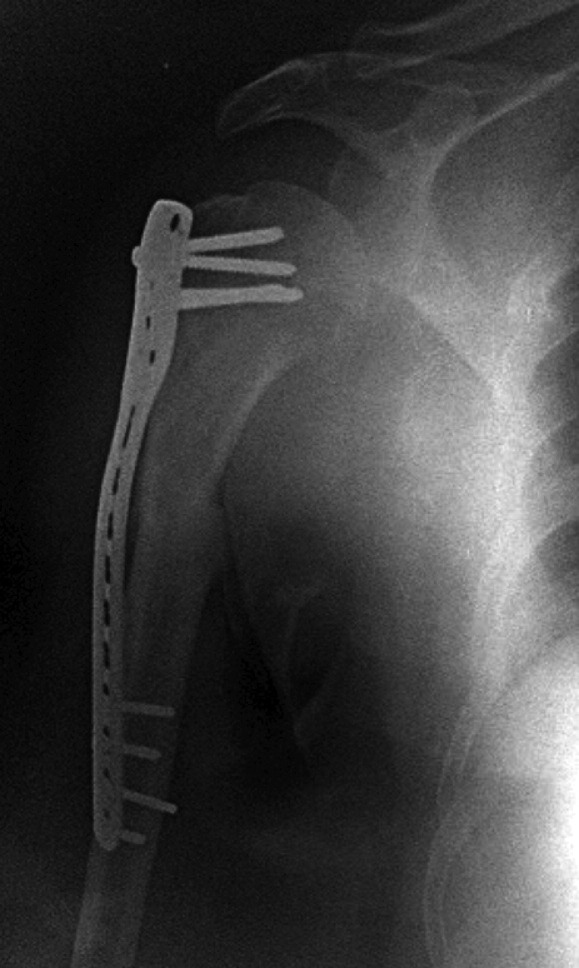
Post-op radiograph at 4 months showing good fracture union with implant *in situ*
